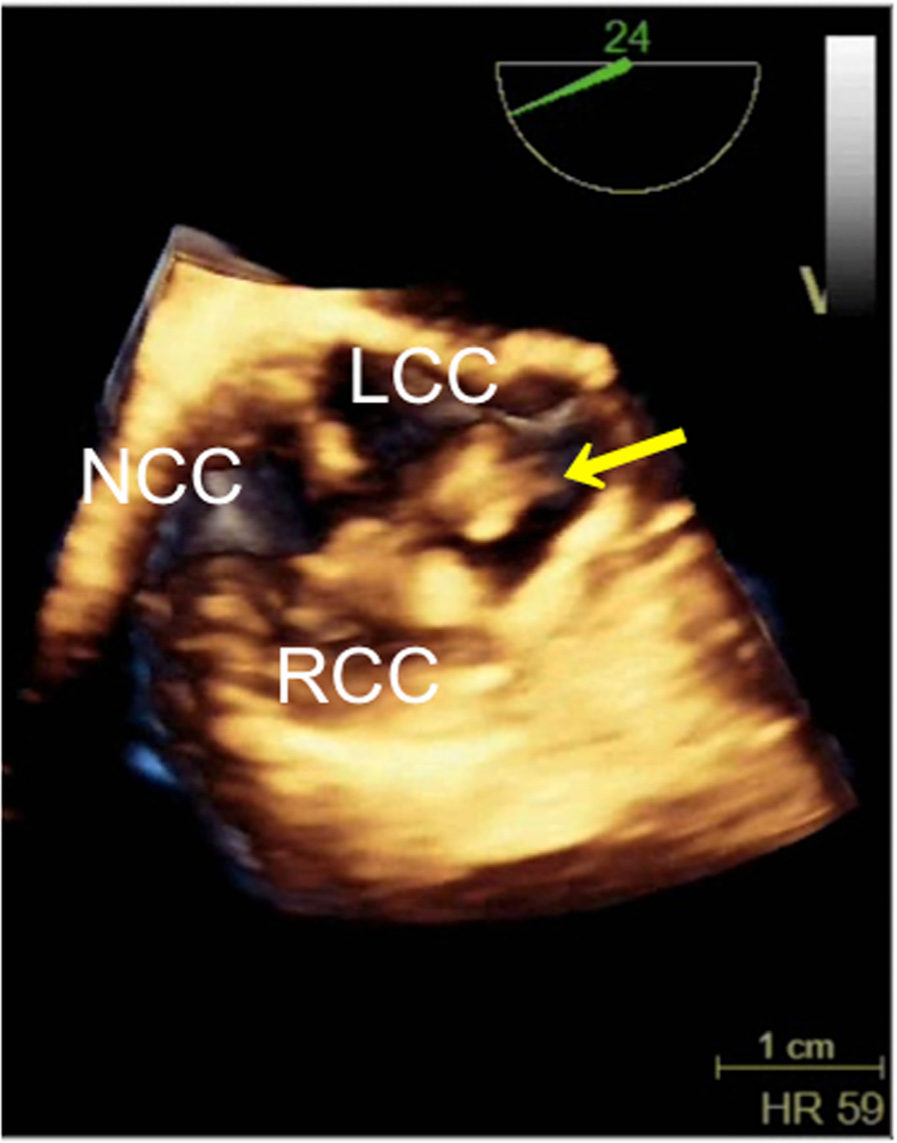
Three-dimensional transesophageal echocardiography image. This short-axis view of the aorta shows a mobile structure fluttering in the sinus of Valsalva of the left coronary cusp (yellow arrow). LCC, left coronary cusp; NCC, non-coronary cusp; RCC, right coronary cusp.
